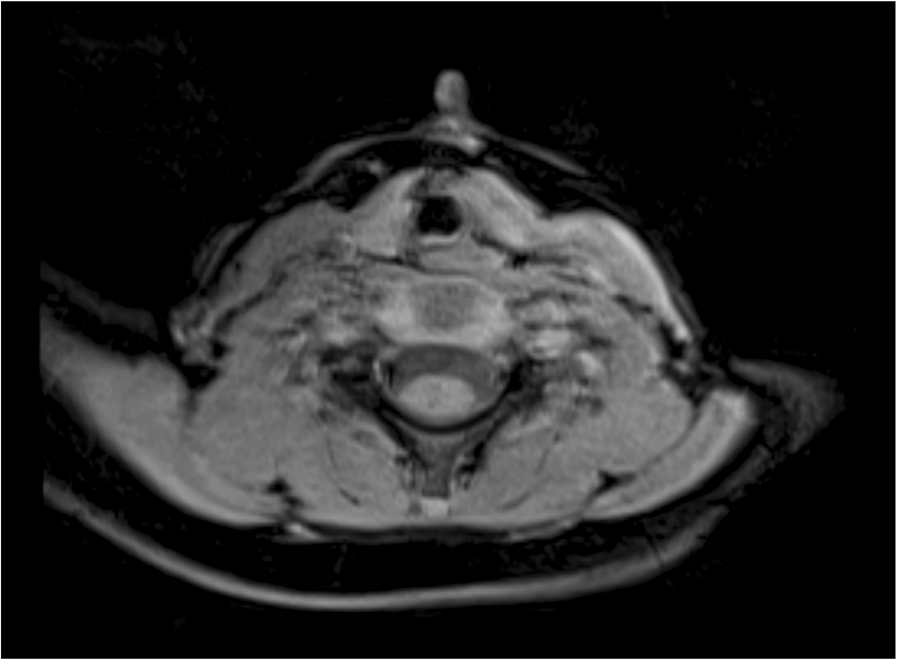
Magnetic resonance imaging view of the patient’s neck, from approximately 1 year prior to the surgery. Note the nipple that belongs to the lesion
